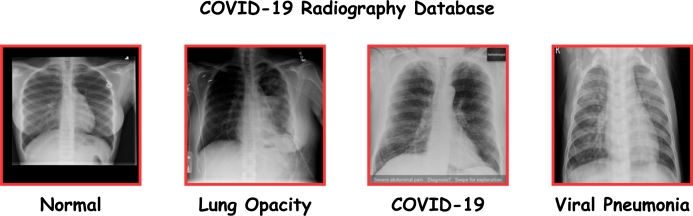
Sample from the “COVID-19 Radiography Database” [66]. From Left: Normal, Lung Opacity, COVID-19, and Viral Pneumonia cases.
